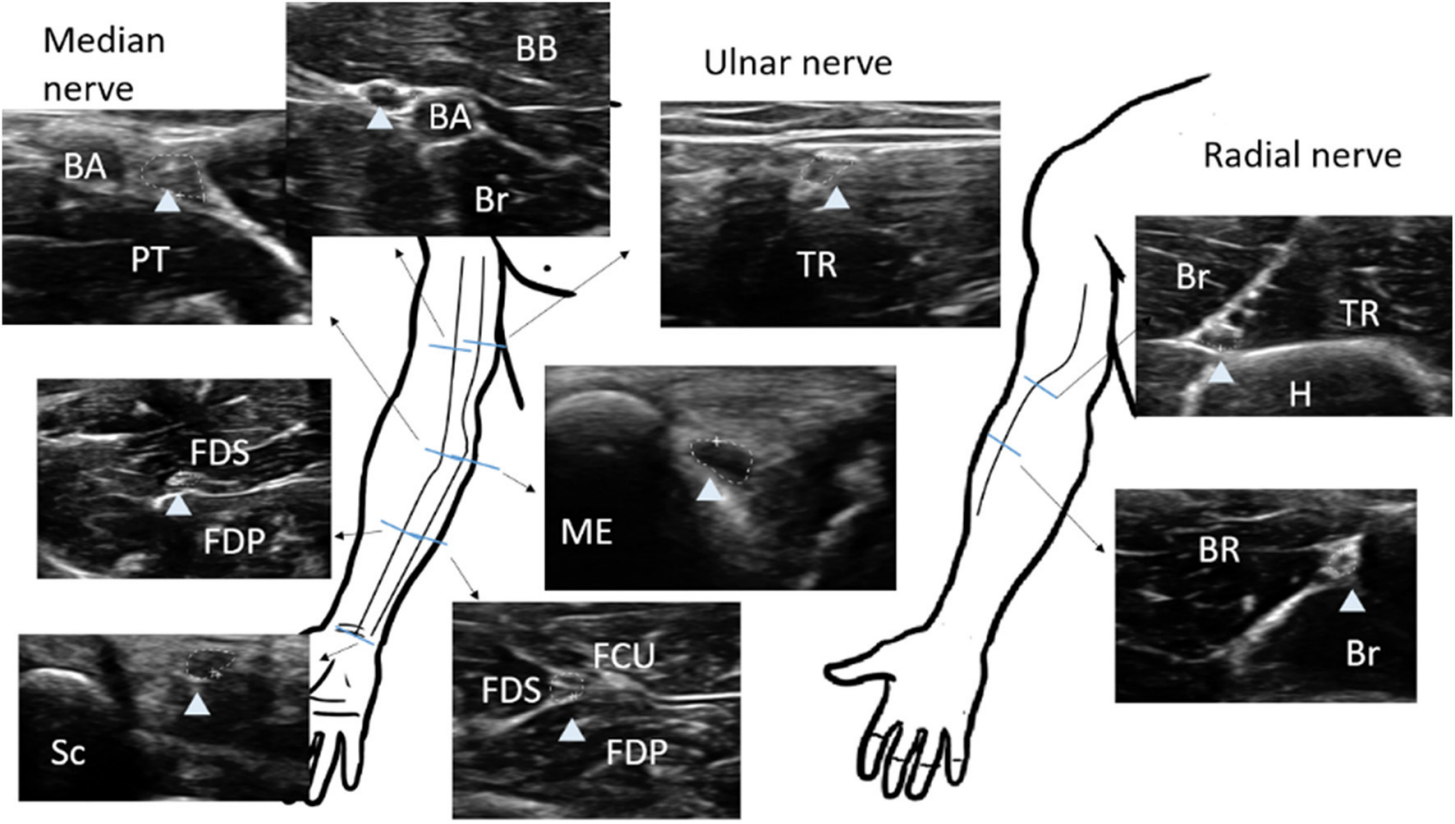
Sites of nerve ultrasonography to determine the cross-sectional area (CSA) in the median, ulnar, and radial nerves. The CSA of each nerve (arrow head) is measured within the hyperechoic rim (dotted line). Median nerve sites: wrist, mid-forearm, antecubital fossa, and mid-arm. Ulnar nerve sites: mid-forearm, cubital tunnel, and mid-arm. BA, brachial artery; BB, biceps brachii; Br, brachialis; FCU, flexor carpi ulnaris; FDS, flexor digitorum superficialis; FDP, flexor digitorum profunuds; ME, medial epicondyle; SB, scaphoid bone; PT, pronator teres; TR, triceps.
